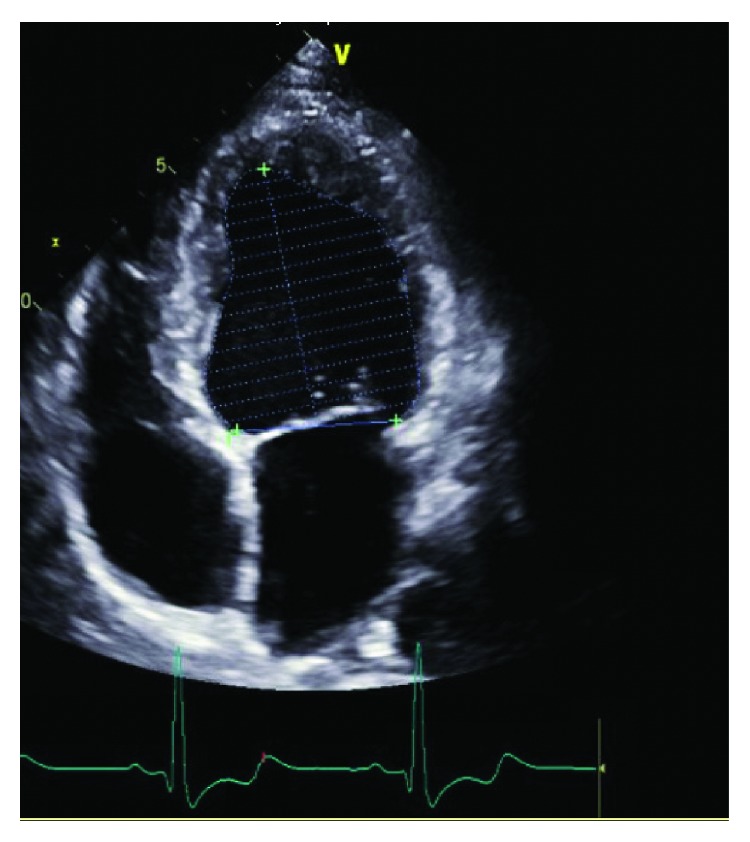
Echocardiogram showing moderate dilation of the left ventricle and mildly reduced ejection fraction.
